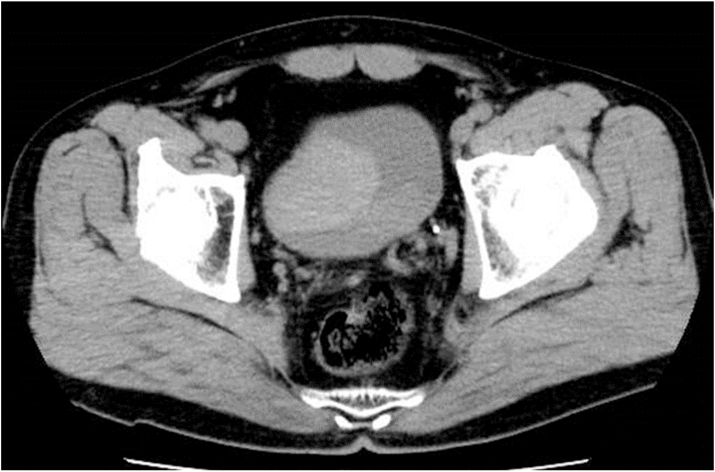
Computed tomography (CT) showed a mass near the bladder right sidewall, and massive blood clots in the bladder.
